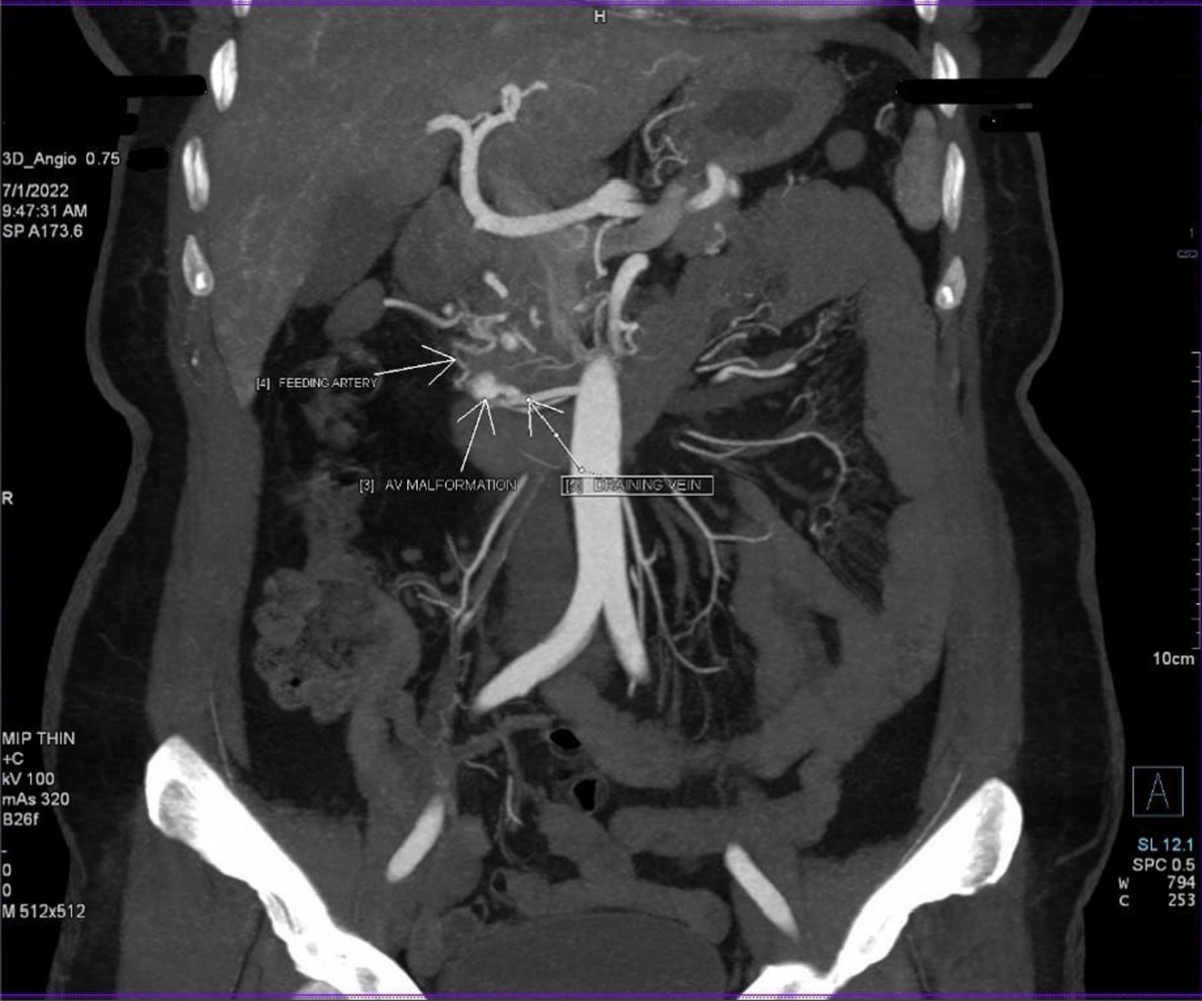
Computed tomography angiogram showing AVM with feeding artery as superior mesenteric artery, nidus around uncinate process, and draining to superior mesenteric vein.
